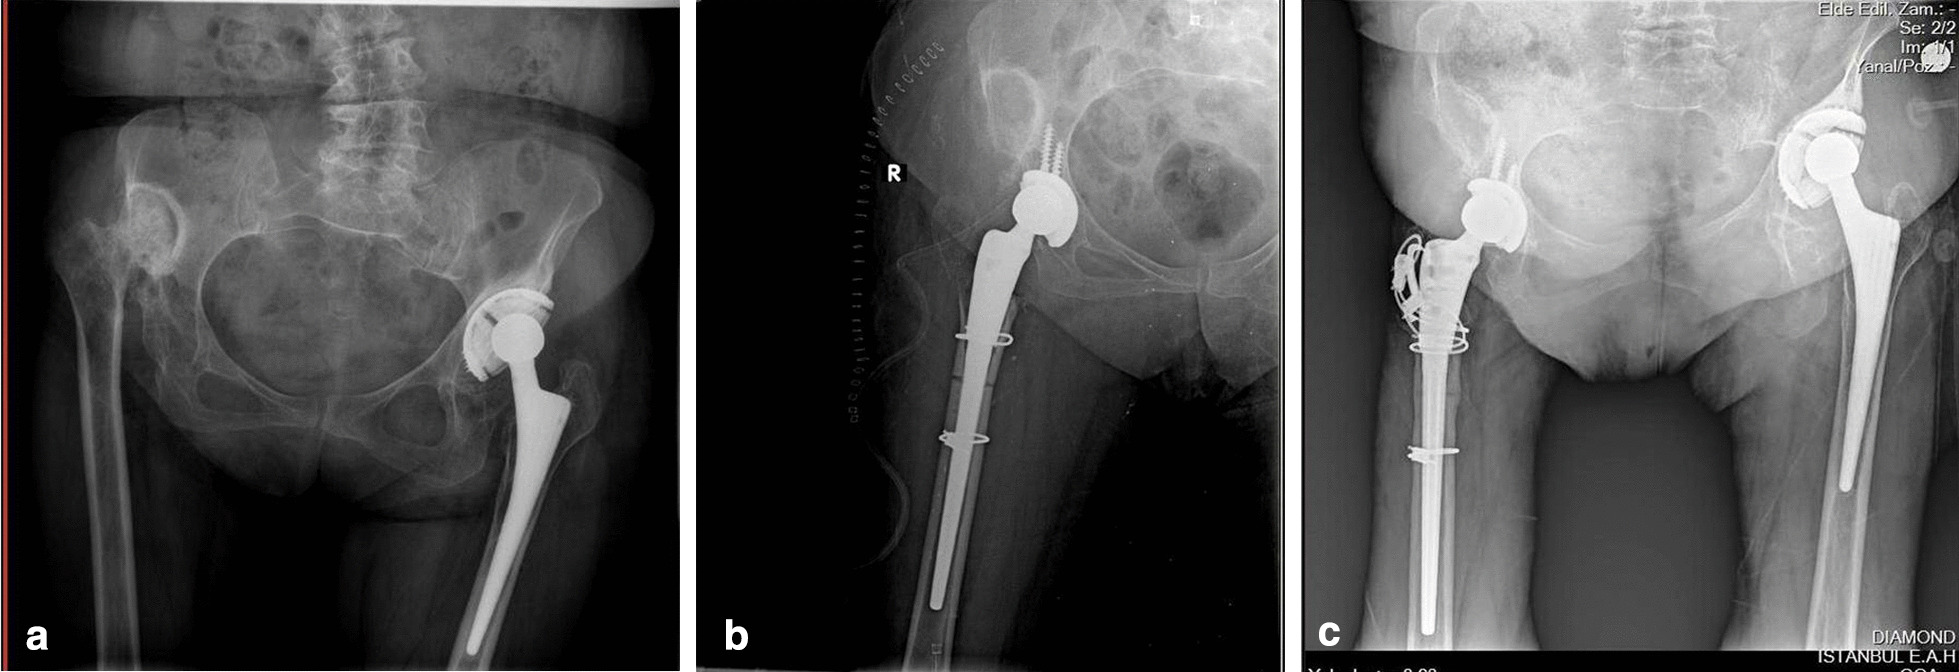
Radiographs of a 53 years old female with high hip dislocation. a Preoperative anteroposterior view. b Early postoperative radiographic image. Hip was reconstructed at the level of the anatomic hip center by cementless modular total hip arthroplasty combined with transverse osteotomy. Trochanter major fracture was observed and treated with trochanteric plate and cable grip, with bony union achieved at the 8th postoperative week (c)
